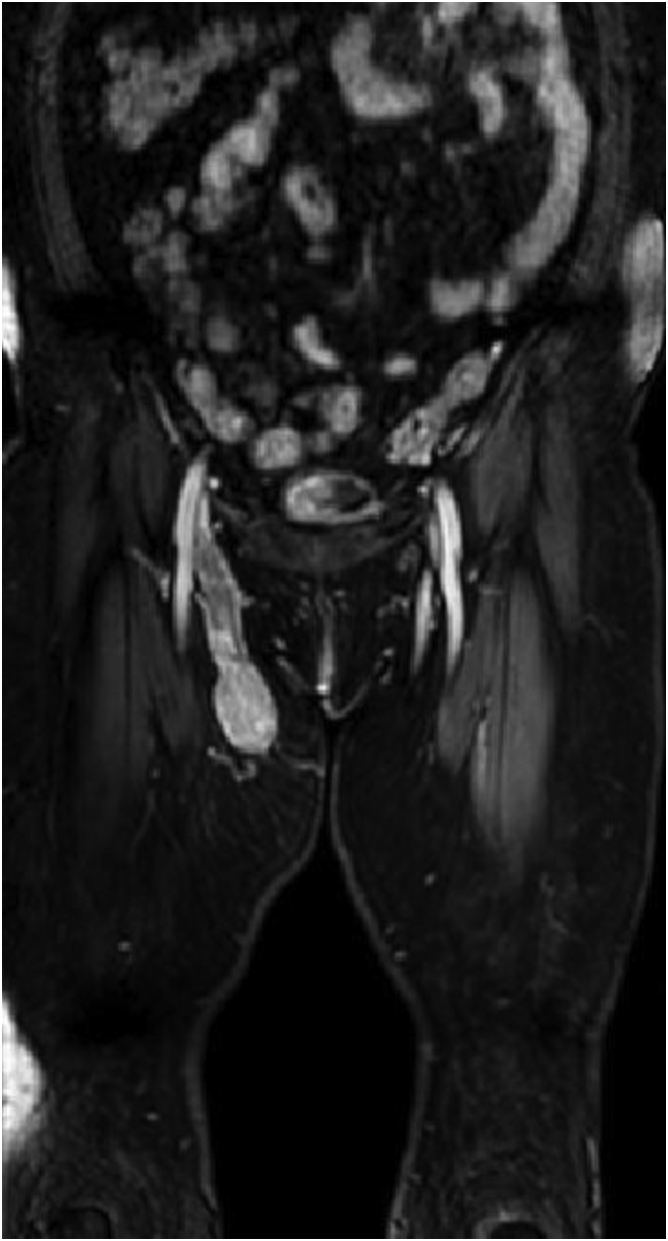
Coronal view of contrast-enhanced magnetic resonance imaging (MRI) showing an inhomogeneously enhancing mass within the proximal great saphenous vein (GSV) expanding the lumen. Less prominent enhancement is present in its superior portion, mimicking a filling defect and thrombosis.
